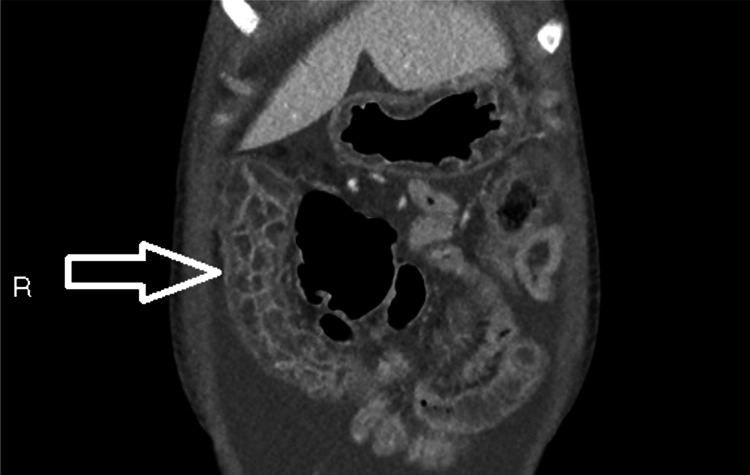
Contrast-enhanced CT of the abdomen and pelvis in the sagittal plane showing transverse colitis The transverse colon (arrow) demonstrates inflammatory colitis; the bowel wall and segmental haustra are thickened and enhanced by hyperaemia. There is significant oedematous change within the mural and submucosal layers, in addition to intra-luminal fluid filling, creating the characteristic appearance of transverse colitis. This figure underscores that lupus enterocolitis can present in various segments of the GI tract.
